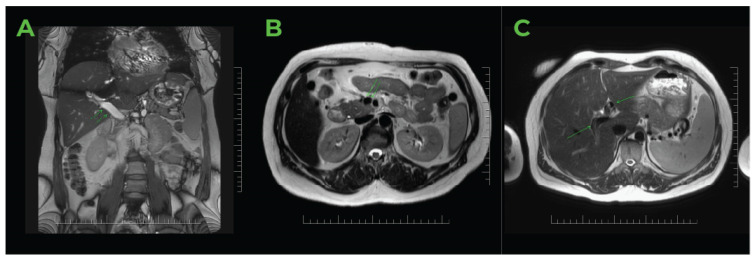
Control abdominal MRI study 15 months after delivery. (A) The coronal plane BTFE sequence showed renewed flow signals in the previously thrombosed portal vein and its branches within the liver, confirming complete recanalisation. (B,C) The axial plane T2 confirmed the normalisation of the lumen width and the flow signals of the superior mesenteric vein along with the branches of the portal vein in the liver. *Source*: MJ.
